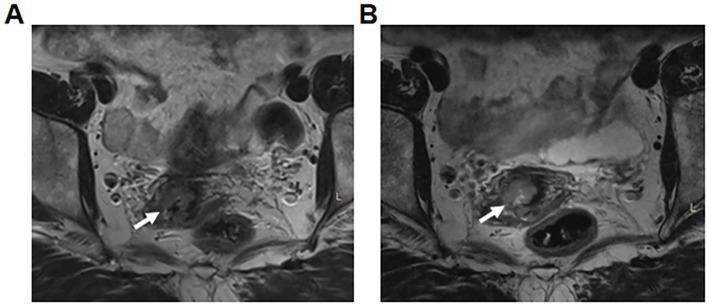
T2-weighted imaging (T2WI) of stage IB1 cervical cancer. (A) Abdominal coil T2WI sequence. The arrow indicates the lesion. Image clarity score: 3.7. (B) Vaginal coil T2WI sequence in the same patient. The arrow indicates the same lesion, which appears clearer and with improved contrast compared to (A). Image clarity score: 4.8.
